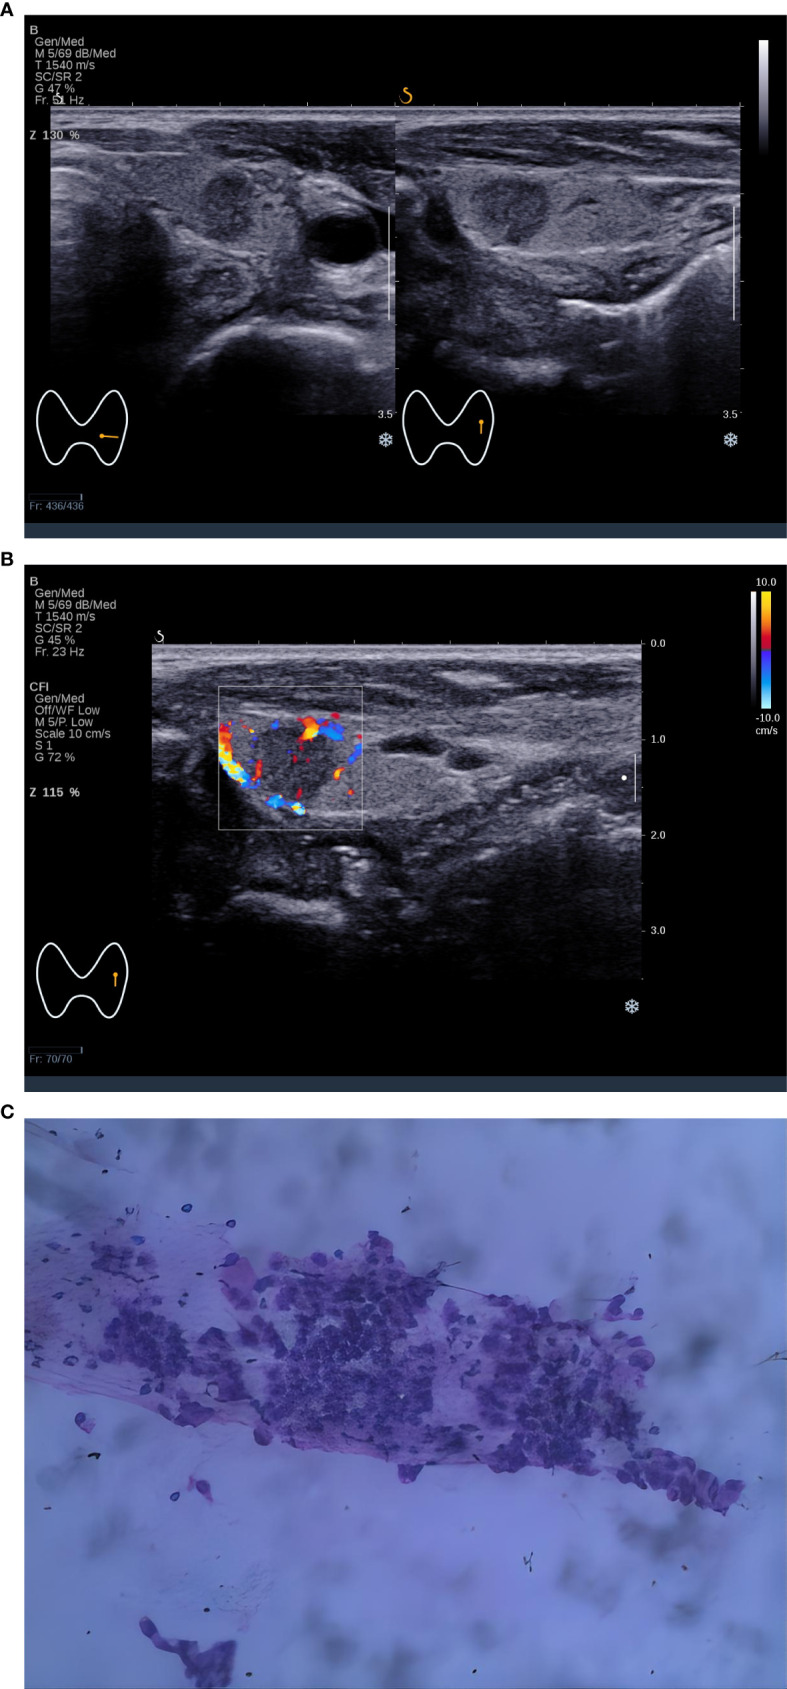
A 5×5 mm thyroid nodule in the left lobe of a 44-year-old woman. (A) Two-dimensional ultrasound of transverse and longitudinal sections showed a nodule with homogeneous echotexture, regular shape, posterior echo unchanged, and the C-TIRADS category was 4A, (B) Color Doppler ultrasound showed blood flow in the nodule. The predictive value calculated by the logistic regression formula was 0.4114 (> 0.2946), which was considered to be Bethesda III/IV. (C) Cytological pathological examination showed Bethesda III.
